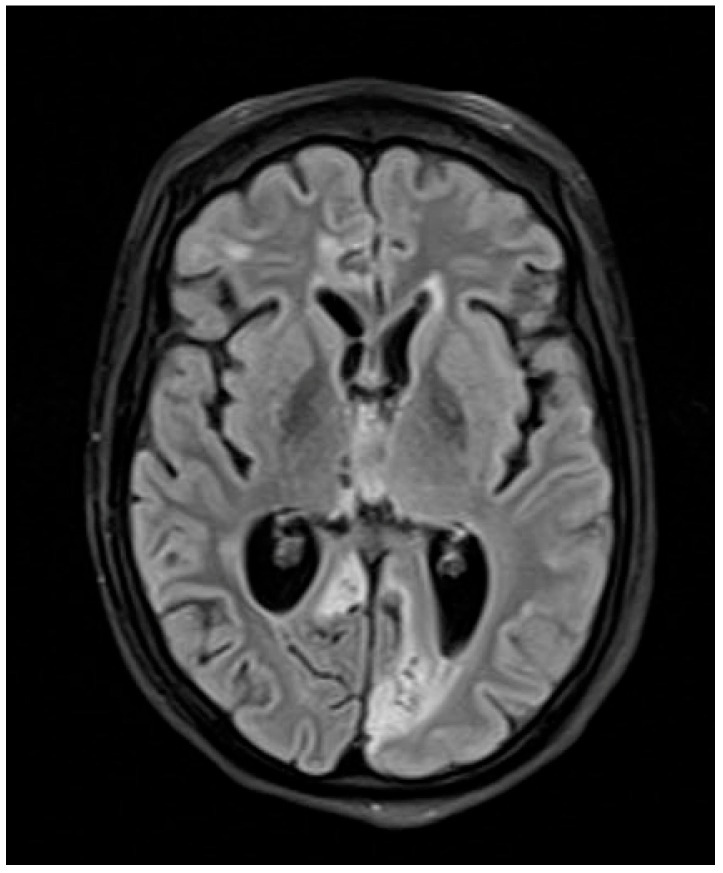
Brain MRI four months after therapy.
